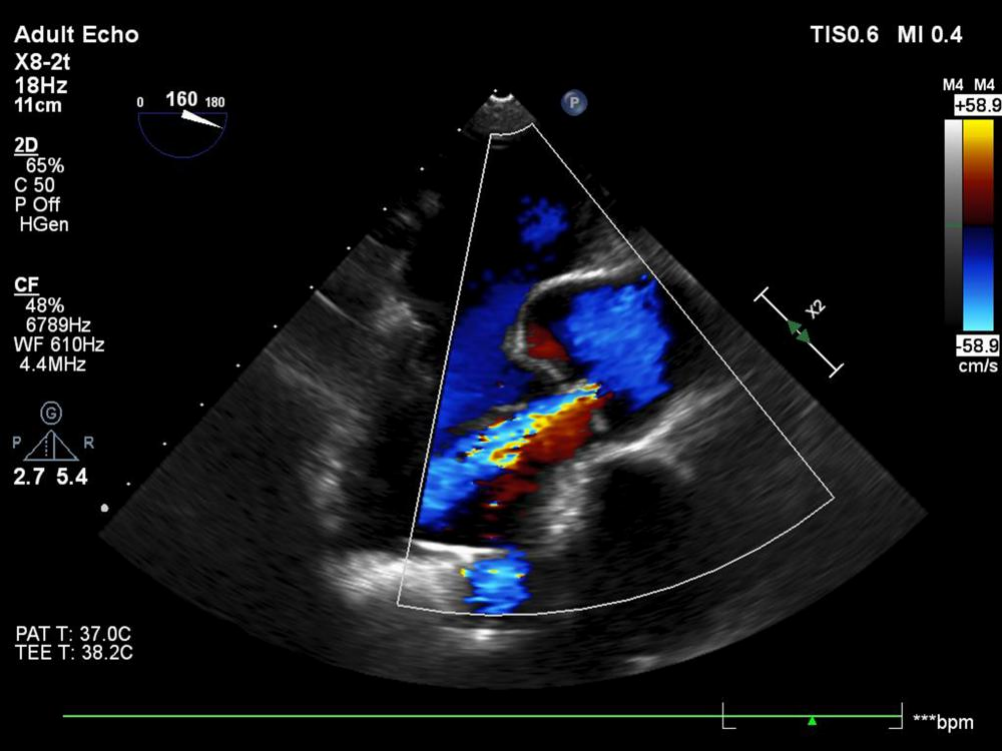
Mid-oesophageal long-axis view on transoesophageal echocardiography, October 2019, demonstrating aortic valve closure and moderate continuous aortic incompetence.
